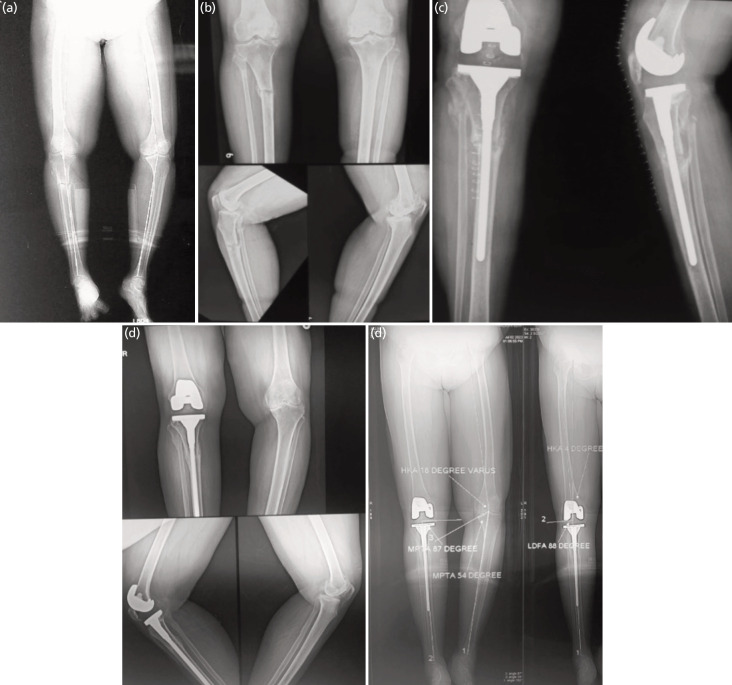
(a) Bilateral lower limb scanogram showing radiological parameters suggesting bilateral varus deformity of knee. (b) Standing antero-posterior and lateral radiograph showing severe osteoarthritis of knee with chronic stress fracture at junction of metaphysis and diaphysis of tibia. (c) Post total knee arthroplasty radiograph showing tibial stem crossing the tibial stress fracture site. (d) Two years post-operative follow-up radiographs showing complete union of tibia with no signs of loosening of implants and fractures. (e) Two years post-operative scanogram showing improved radiological parameters on operated side.
